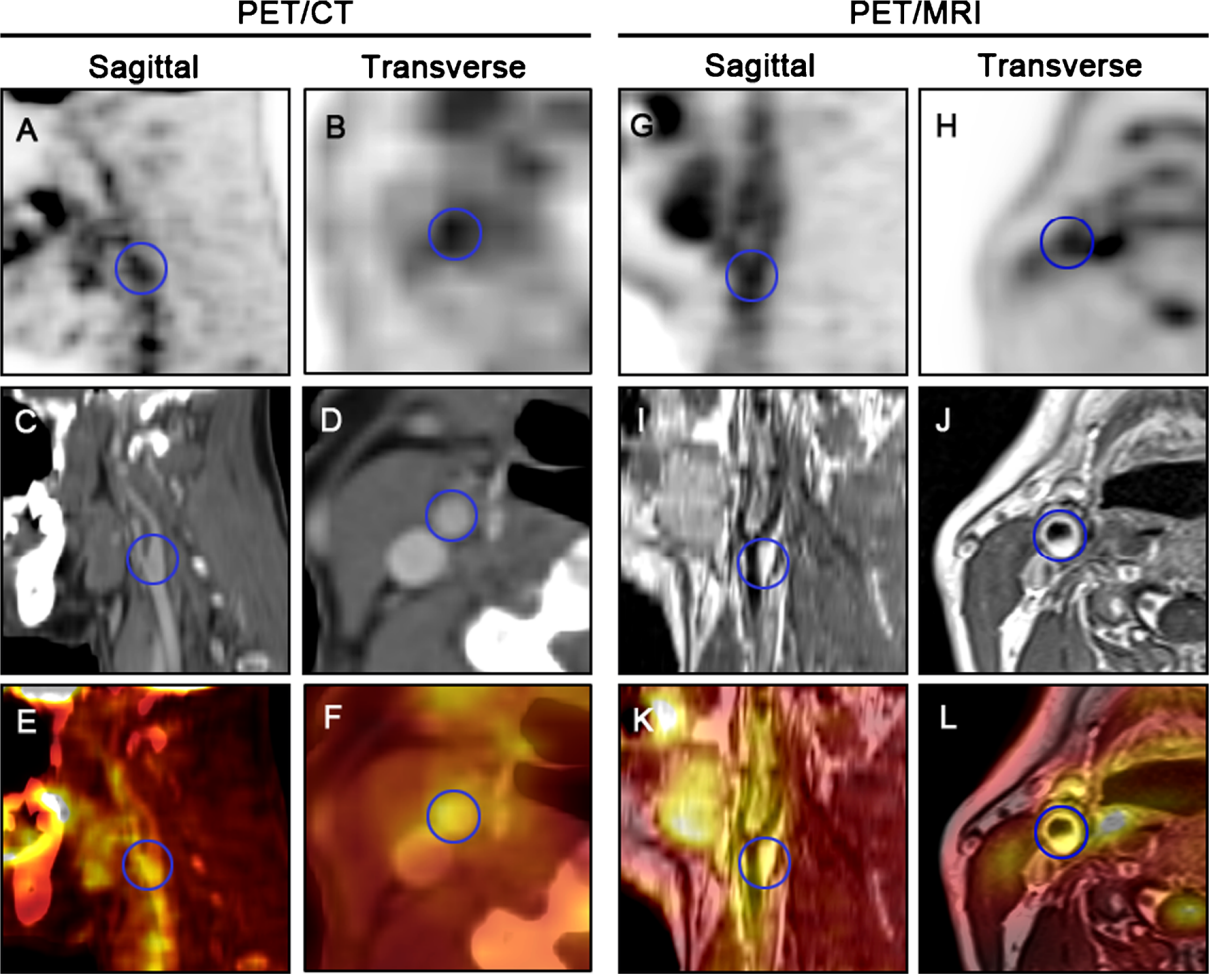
Fused PET/CT (a–f) and PET/MRI (g–l) images of the right carotid artery in a 52-year-old patient with a head and neck tumour (*blue circles* ROIs of a plaque at the carotid bifurcation). The right carotid artery shows focal pathological ^18^F-FDG uptake on the PET images with CT-based attenuation correction. Colocalized focal uptake of ^18^F-FDG is seen on the PET image with MR attenuation correction
